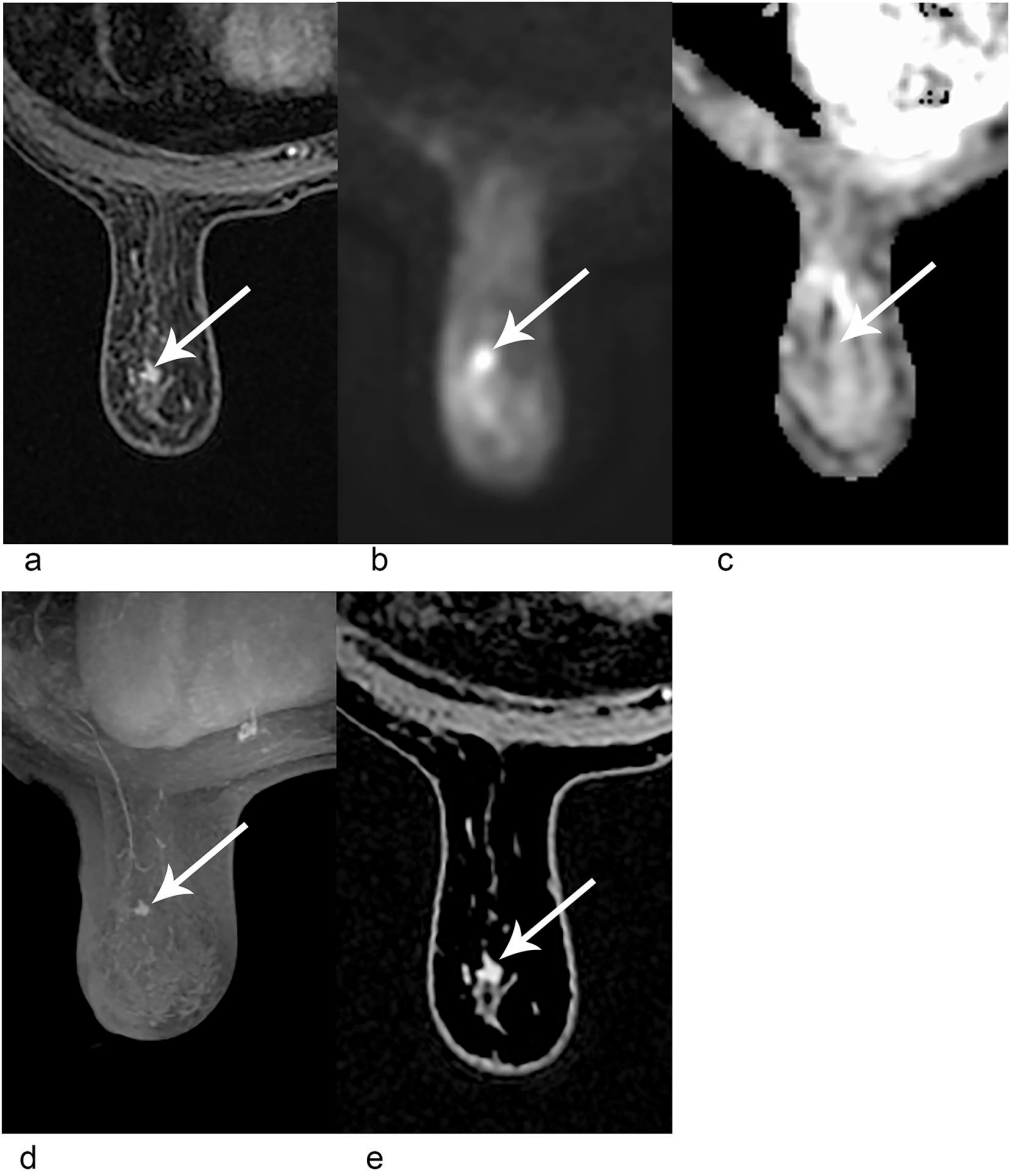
A 50-year-old female; an invasive ductal carcinoma was not found on mammography with the background of heterogeneously dense breasts. (1) Unenhanced (UE)-MRI including images of FS-T2WI (a), DWI (b), and ADC map (c). An irregular mass of 5 mm diameter in the left breast (arrow) was detected by FS-T2WI. In the DWI sequence, the mass presented hyperintensity with corresponding ADC value = 1.61 × 10^–3^ mm^2^/s. As the result of the ADC value was higher than the cutoff, one of the readers scored this lesion as benign, whereas the other one thought this mass was malignant, mainly due to the irregular shape. (2) Abbreviated protocol (AP)-MRI consisting of the maximum-intensity projection (MIP) (d) and first post-contrast subtracted (FAST) (e). The images showed an enhanced mass with a slightly lobulated margin on the left breast, which was classified as BI-RADS 4.
